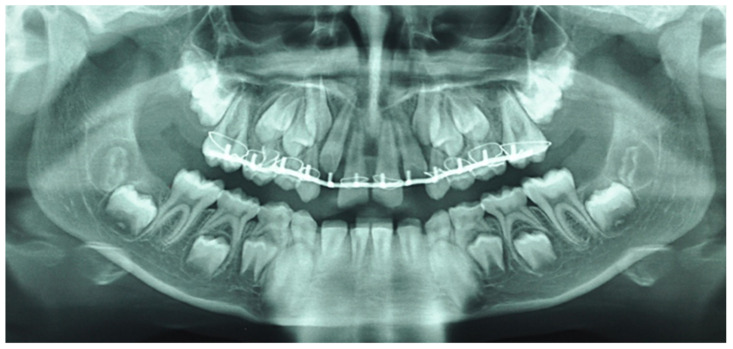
Orthopantomogram of the patient’s initial dental condition in October 2015.
